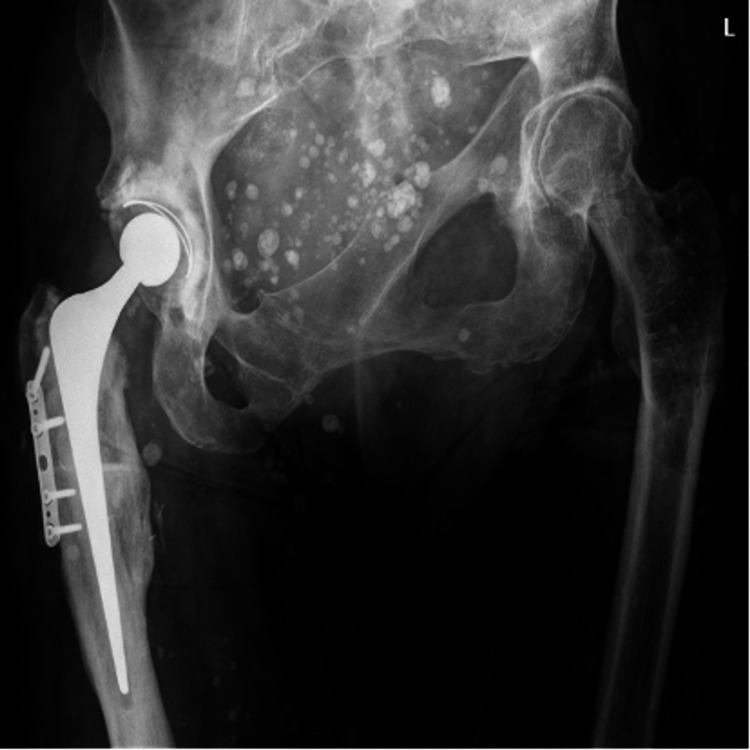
Frontal radiograph showing the extensively deformed pelvis and right total hip arthroplasty.
